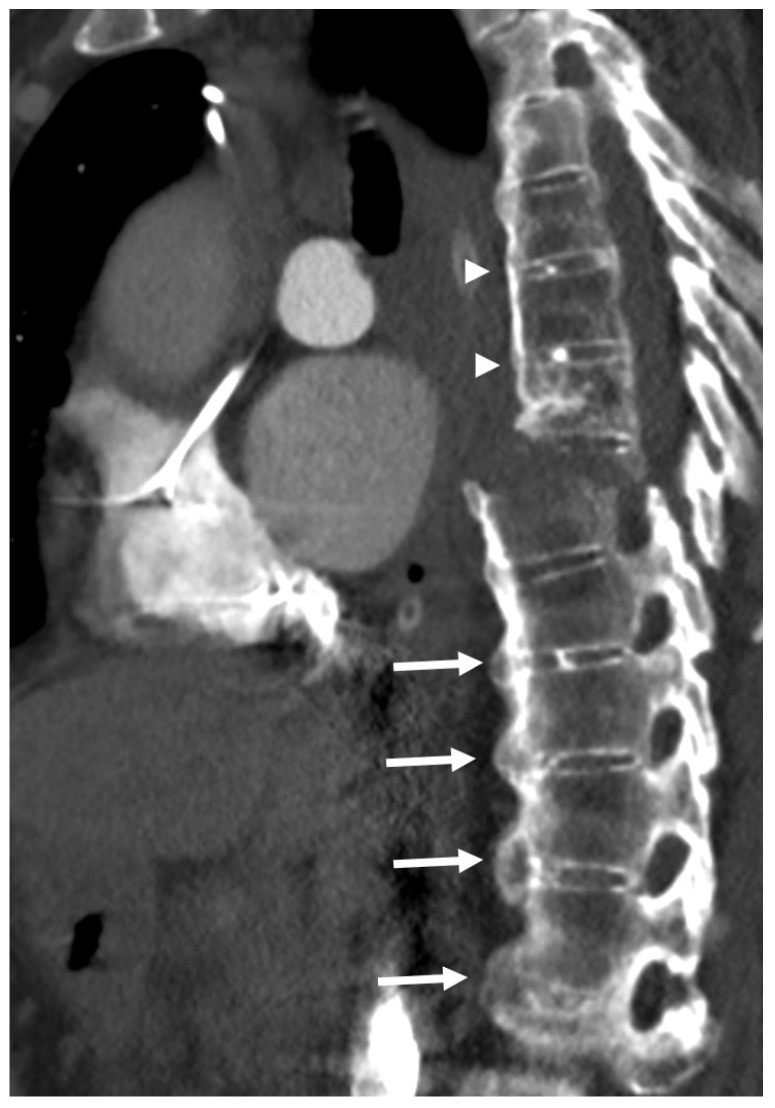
Sagittal CT reconstruction of the thoracic spine of a 78-year-old patient with thoracic DISH after low-energy trauma, showing an unstable extension-type fracture of the mid-thoracic spine. In the given slice, there are two vertically oriented bony bridges in the upper thoracic spine (arrowheads) that may lead to the misconception that this is a patient with ankylosing spondylitis; however, there are clearly many flowing osteophytes compatible with DISH in the lower part of the thoracic spine (arrows).
